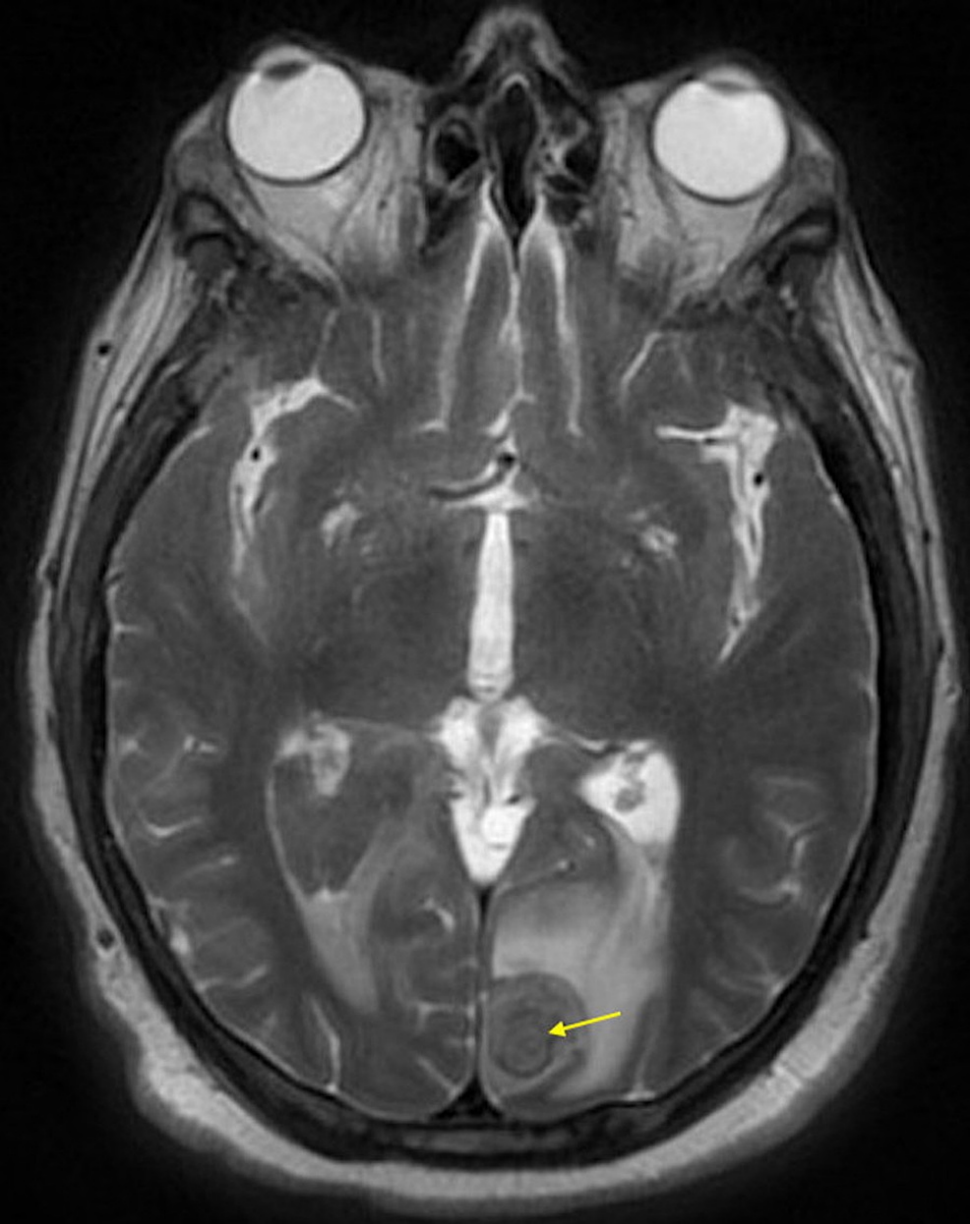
Brain magnetic resonance imaging (MRI) demonstrating focus of acute parenchymal hemorrhage in the left occipital lobe measuring 2.4 x 1.8 x 1.8 cm and an enhancing nodule in that region measuring 1.1 x 1.1 cm (arrow).
